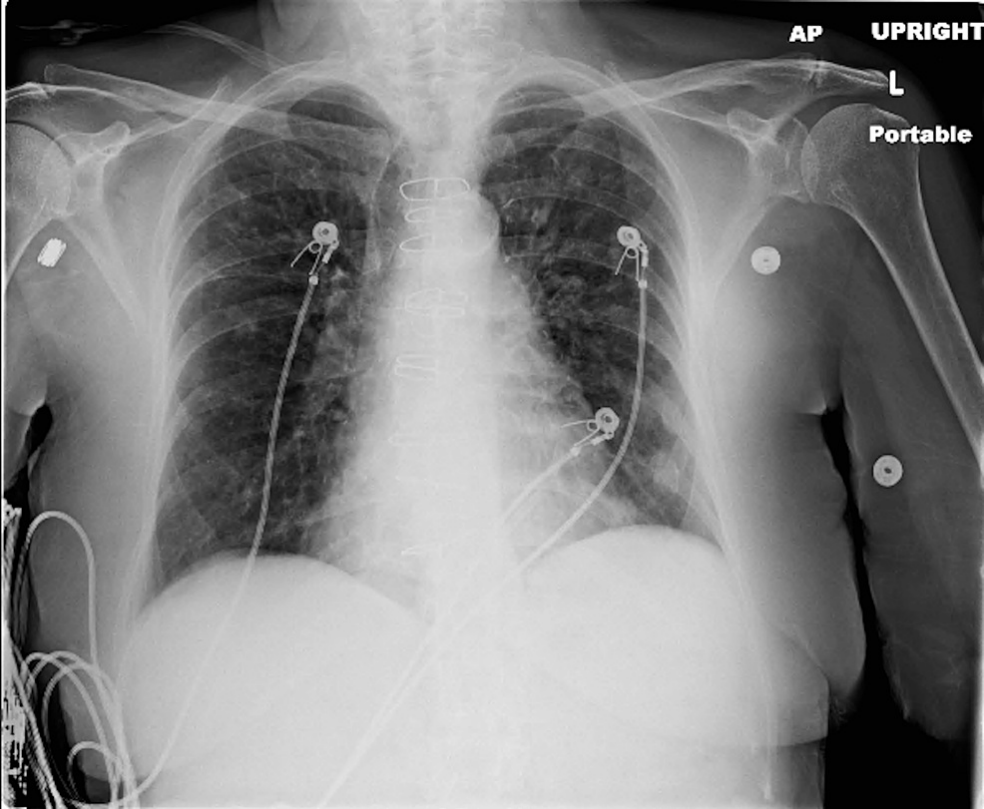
Initial chest X-rays were significant for patchy ground-glass opacifications.
